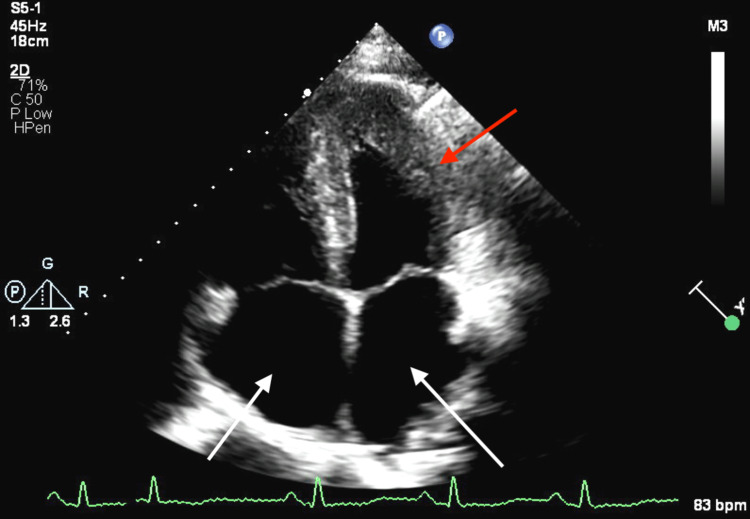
Apical four-chamber view demonstrating left ventricular hypertrophy and biatrial enlargement The red arrow points to left ventricular hypertrophy; the white arrows point to biatrial enlargement.
